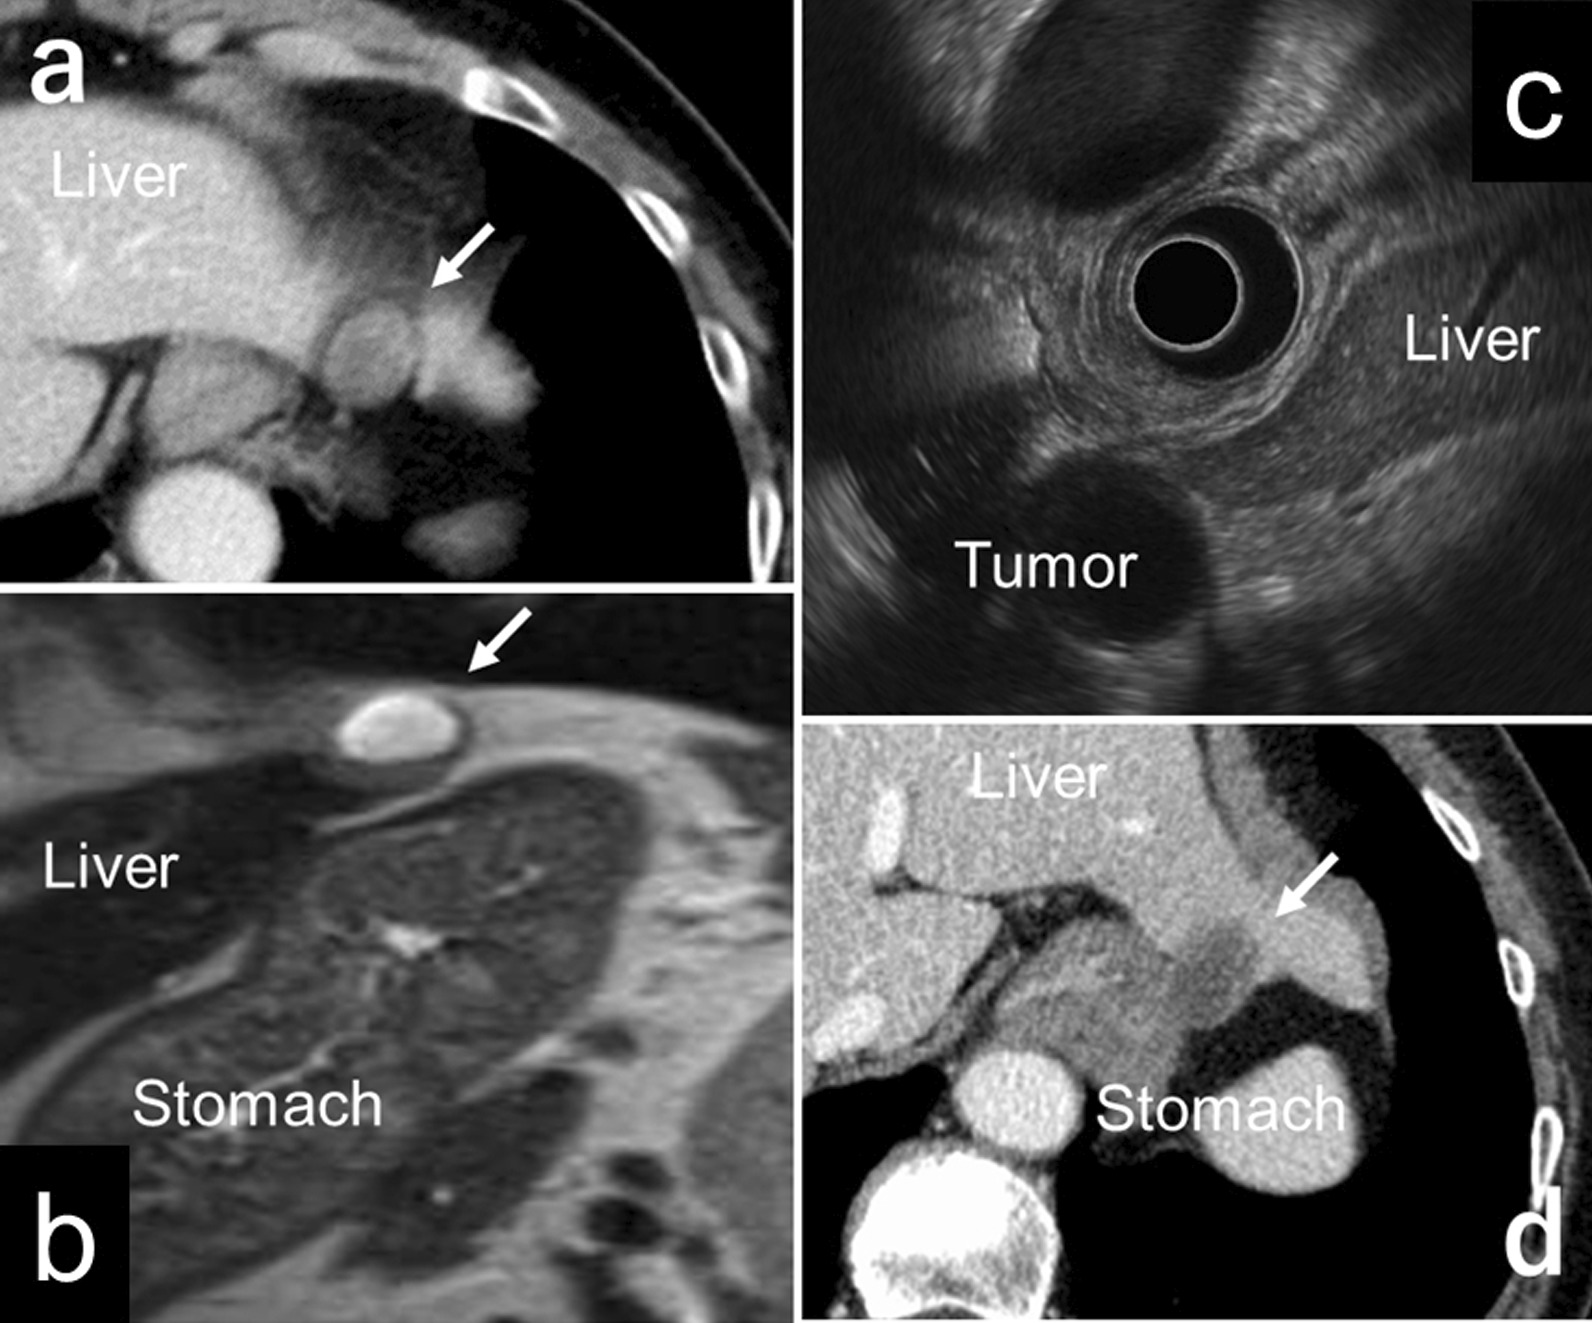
Preoperative imagings. a Computed tomography on admission to previous hospital showed that there was a low density, round mass, measuring approximately 20 mm in diameter, located between the upper gastric body and left lateral segment. b T2-weighted magnetic resonance imaging on admission to previous hospital showed a high signal intensity mass, which seemed to be distinct from the stomach. c The latest endoscopic ultrasonography suggested that the tumor was distinct from the stomach and the liver. d The latest computed tomography showed an unclear part of the boundary between the tumor and the gastric wall
